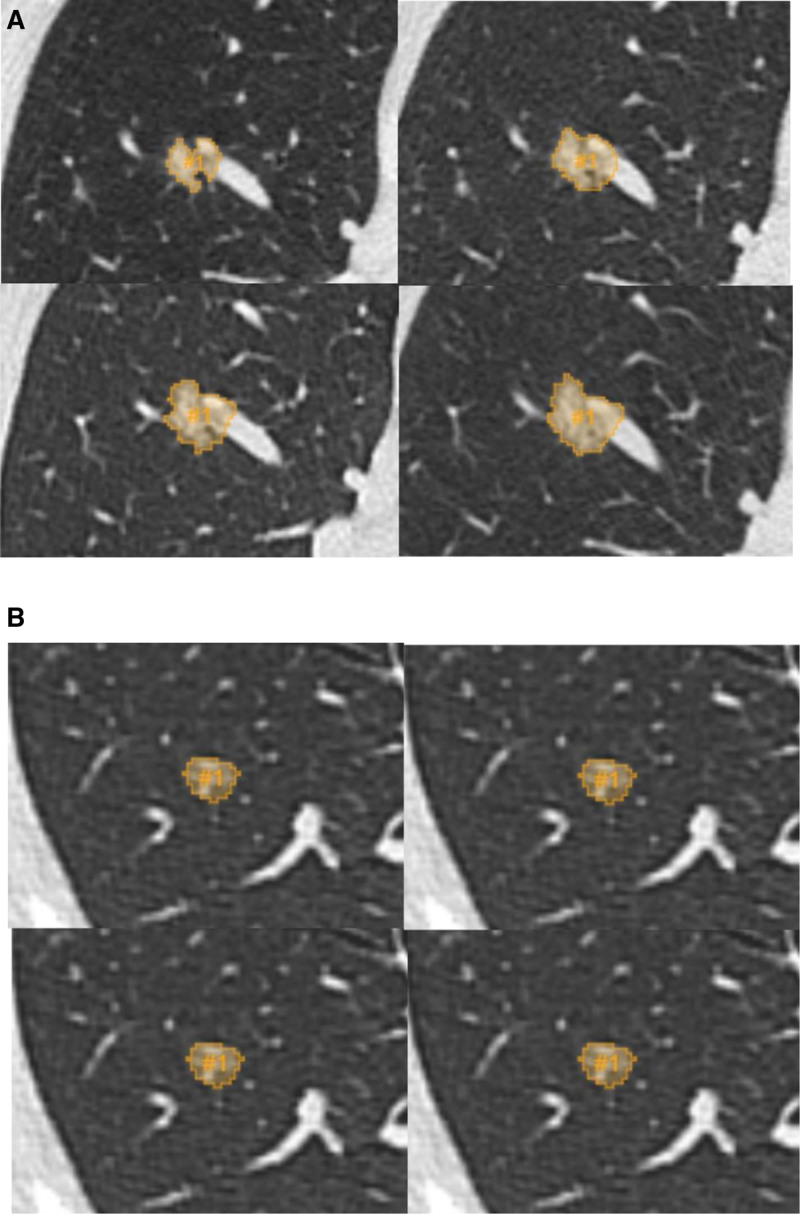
Representative CT segmentation images of ROIs for (A) growing and (B) non-growing subsolid nodule during preoperative follow-up. CT = computed tomography, ROI = region of interest.
